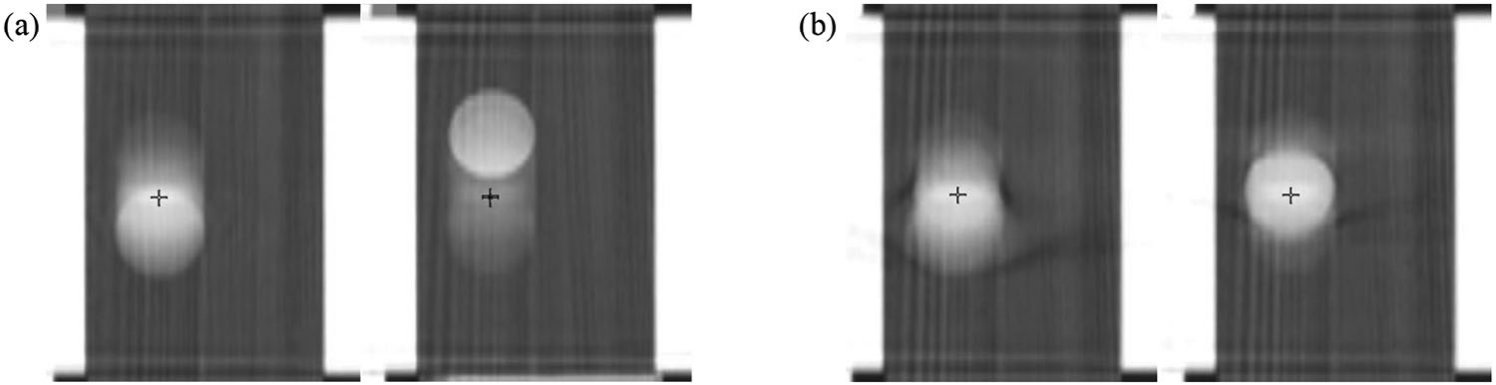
Variations of target location in CBCTs for a highly irregular breathing pattern. (a) 60‐second (b) 6‐second CBCTs.
